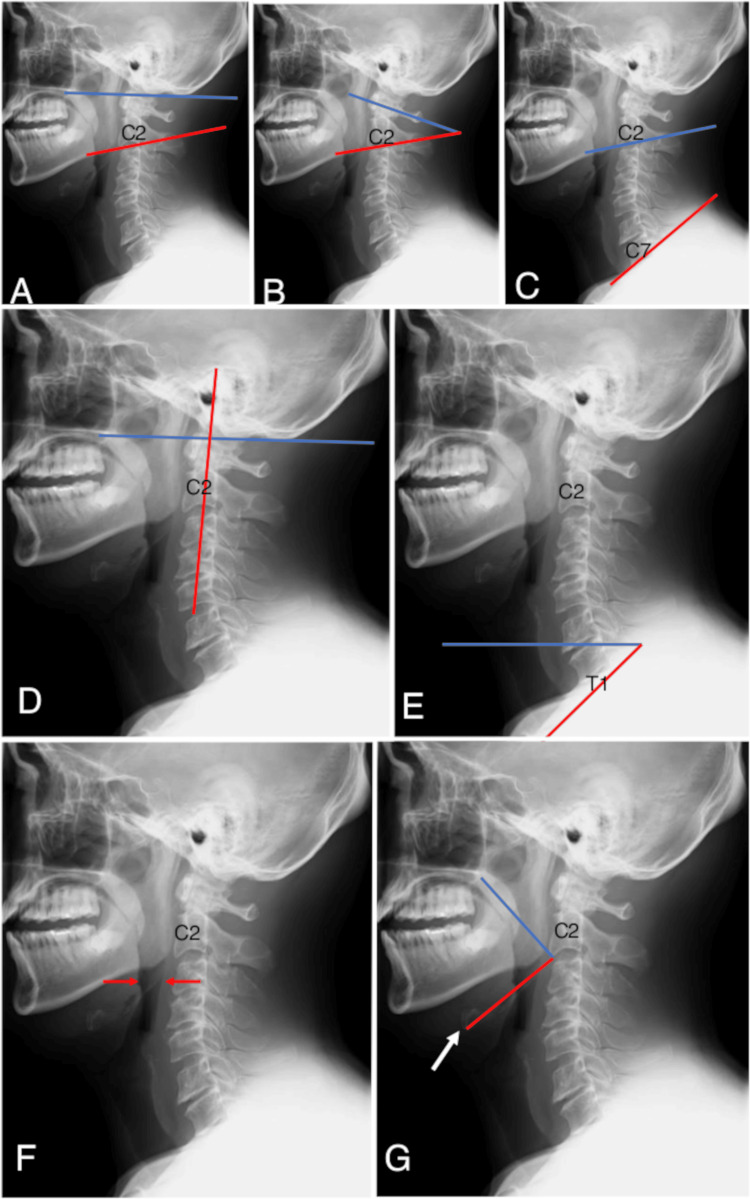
The definition of radiological parameters on X-ray images. (A) The O-C2 angle is defined by the McGregor line (blue line) and a line drawn parallel to the lower endplate of C2 (red line). (B) The C1-C2 angle is defined by a midline penetrating both the anterior arch and posterior arch of C1 (blue line) and a line parallel to the lower endplate of C2 (red line). (C) The C2-C7 angle is defined by a line parallel to the lower endplate of C2 (blue line) and a line parallel to the lower endplate of C7 (red line). (D) The pharyngeal tilt angle is defined by the McGregor line (blue line) and a line penetrating both the center of the pedicle of C2 and the center of the vertebral body at the apex of cervical sagittal curvature (red line). (E) The T1 slope is defined by the horizontal line (blue line) and a line parallel to the upper endplate of T1 (red line). (F) Pharyngeal airway space was defined as the width of the oropharyngeal cavity at the highest level of the epiglottis. (G) The hard palate-C2-hyoid bone angle was defined as the angle between a line from the posterior margin of the hard palate to the anterior margin of the lower endplate of C2 (blue line) and a line from the anterior margin of the lower endplate of C2 to the anterior margin of the hyoid bone (white arrow) (red line).
